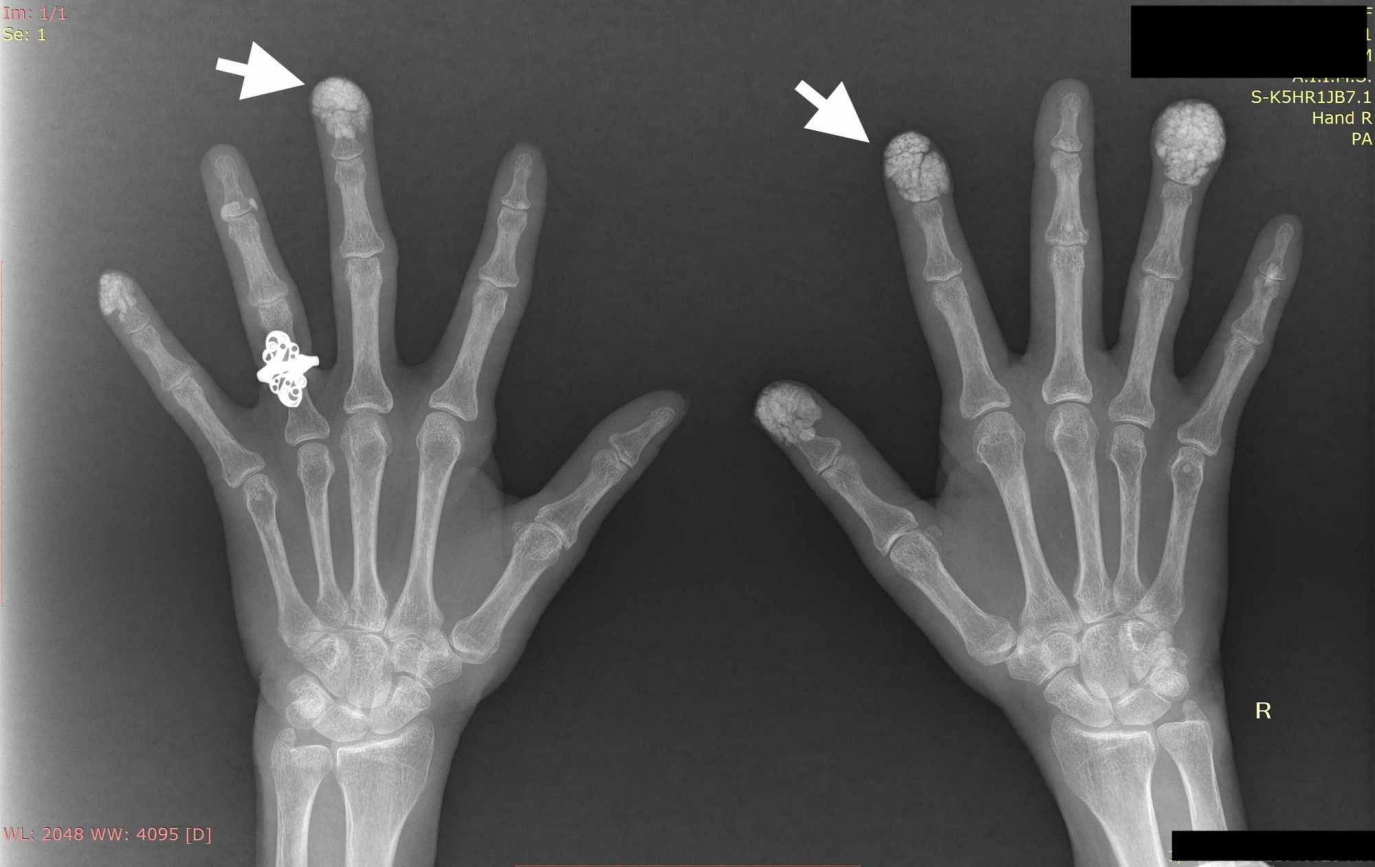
Radiograph of hands showing multiple calcifications within subcutaneous tissue in finger pads (white arrows)
